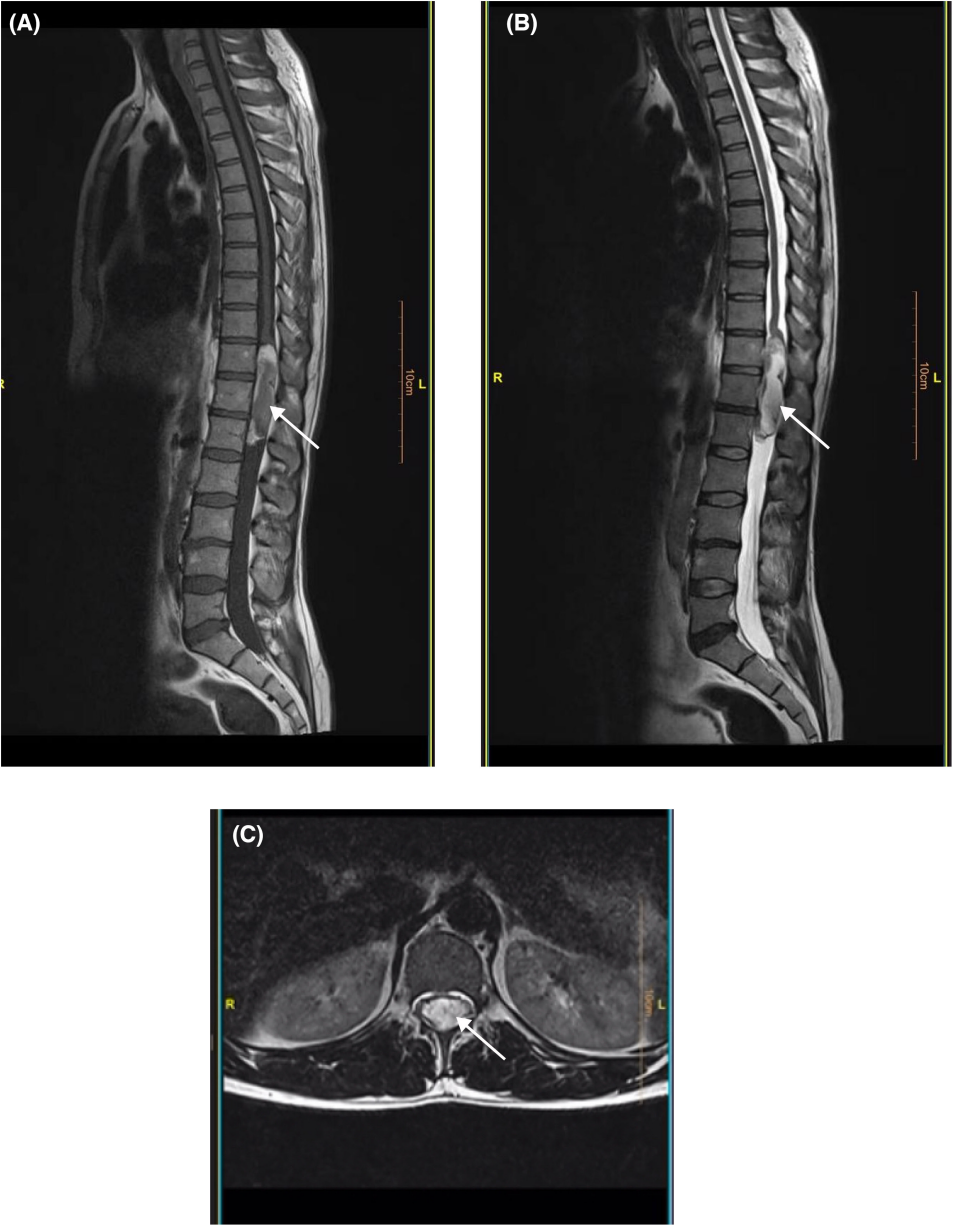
Preoperative sagittal T1‐weighted (A) and T2‐weighted (B) magnetic resonance imaging demonstrate an intradural extramedullary mass lesion at T_11_–L_1_ (conus medullaris). Axial section (C) demonstrates a large‐sized capsular lesion causing spinal cord compression. White arrows point to the tumor location.
